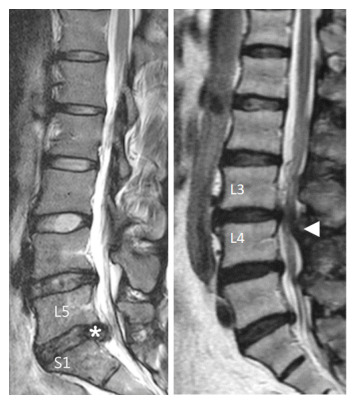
(Left): Preoperative MRI, sagittal view, from a 32-year-old LDH female patient who demonstrated spinal stenosis at the L5–S1 level (left) and a 55-year-old LSS female patient who demonstrated spinal stenosis at the L3–4 level (right). The main cause of stenosis in the LDH patient was from a herniated disc and lesser hypertrophy of the LF. On the other hand, the spinal stenosis in the LSS patient was from severe hypertrophy of the LF. Star: herniated disc. Arrowhead: hypertrophy of LF.
